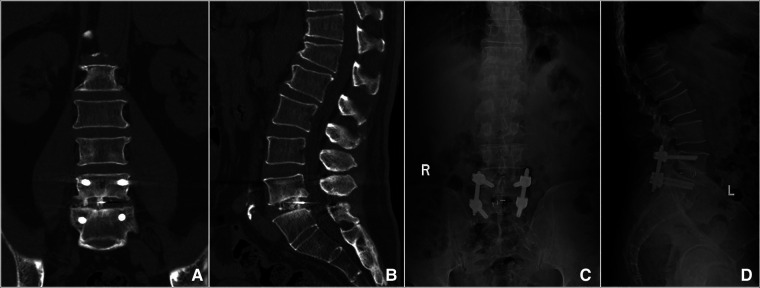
Imaging findings during postoperative follow-up. (A,B) Coronal and sagittal CT showed that the cage was well positioned and high-density bone fusion between vertebral bodies at 6 months postoperatively. (C,D) A 14-month postoperative x-ray showed bony fusion and the instrumentation was in a good position.
